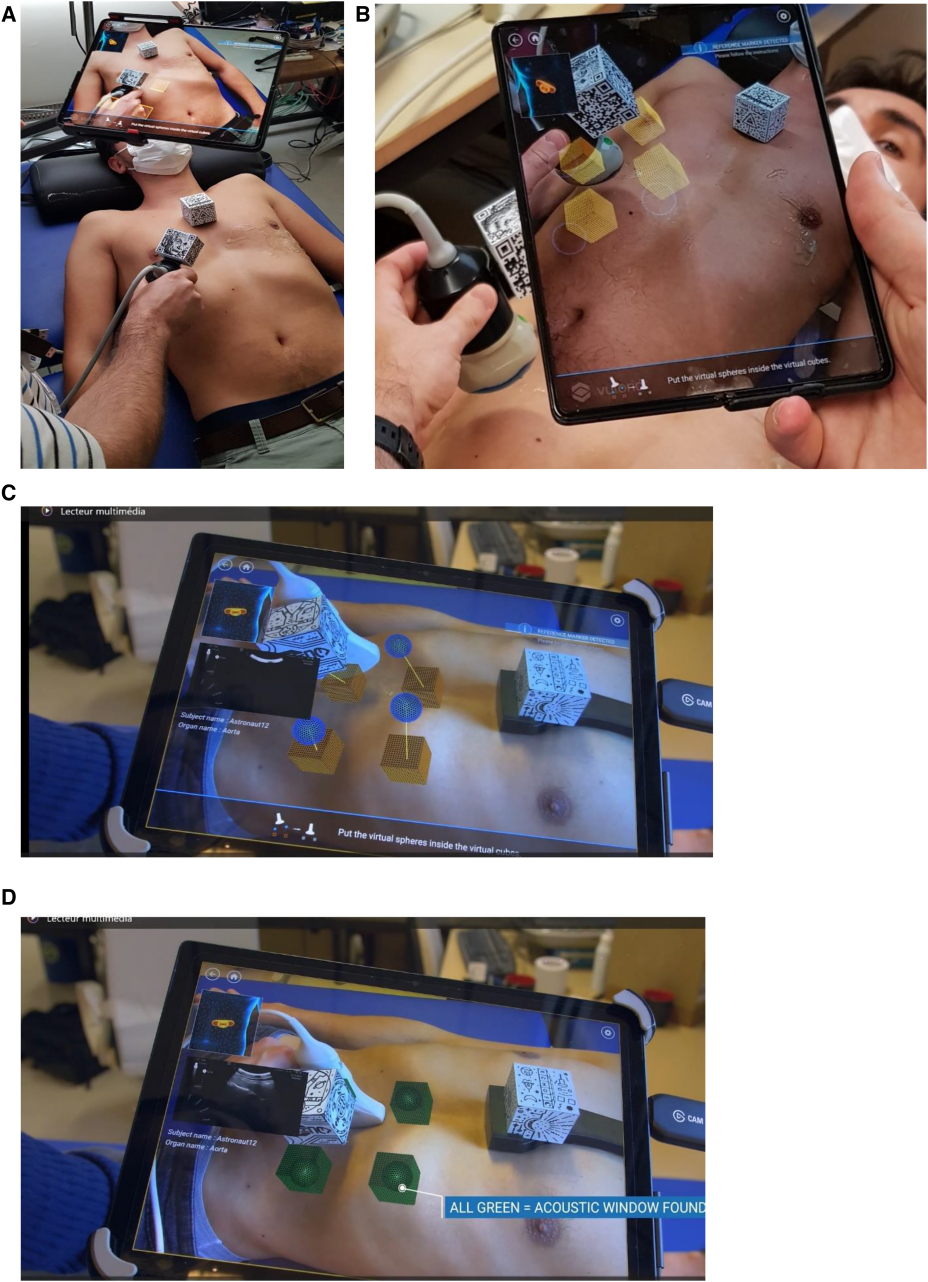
(A,B) View of the setup: QR cube fixed on the ultrasound probe body and on the chest support, and the Echo-QR software running on a smartphone/tablet placed on an articulated arm. During the acquisition phase, an expert saves probe positioning data. The relative position of the two QR cubes is displayed, corresponding to the four cubes on the tablet screen. During the restitution phase (C,D), virtual cubes indicate the probe positioning previously saved by the expert sonographer, and spheres indicate the current position of the probe. The novice operator moves the probe until the four spheres align with the four corresponding cubes and turn green, indicating proper alignment and orientation (C,D). The echographic image of the organ appears on the smartphone/tablet.
